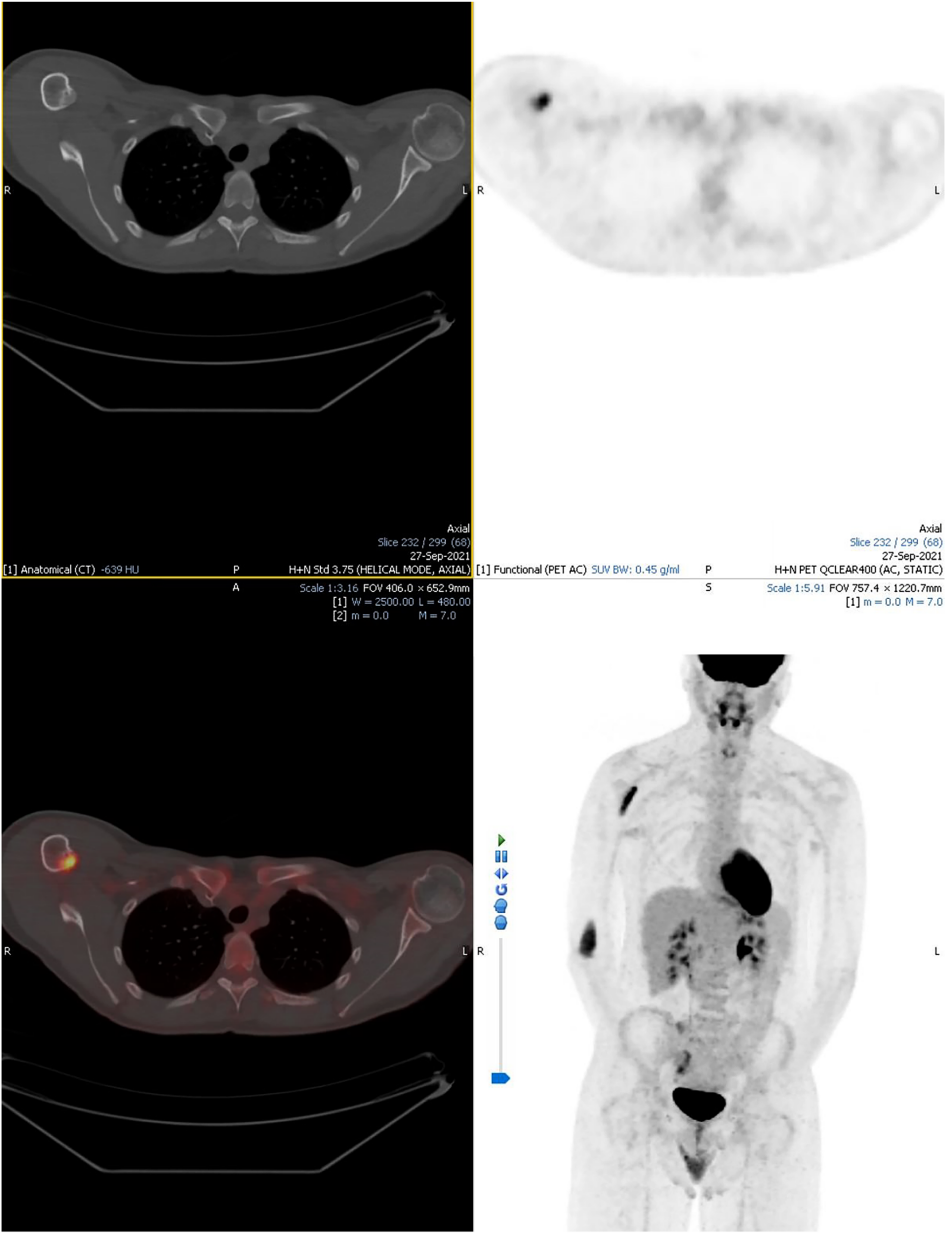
PET‐ CT following 2 cycles of ABVD.
